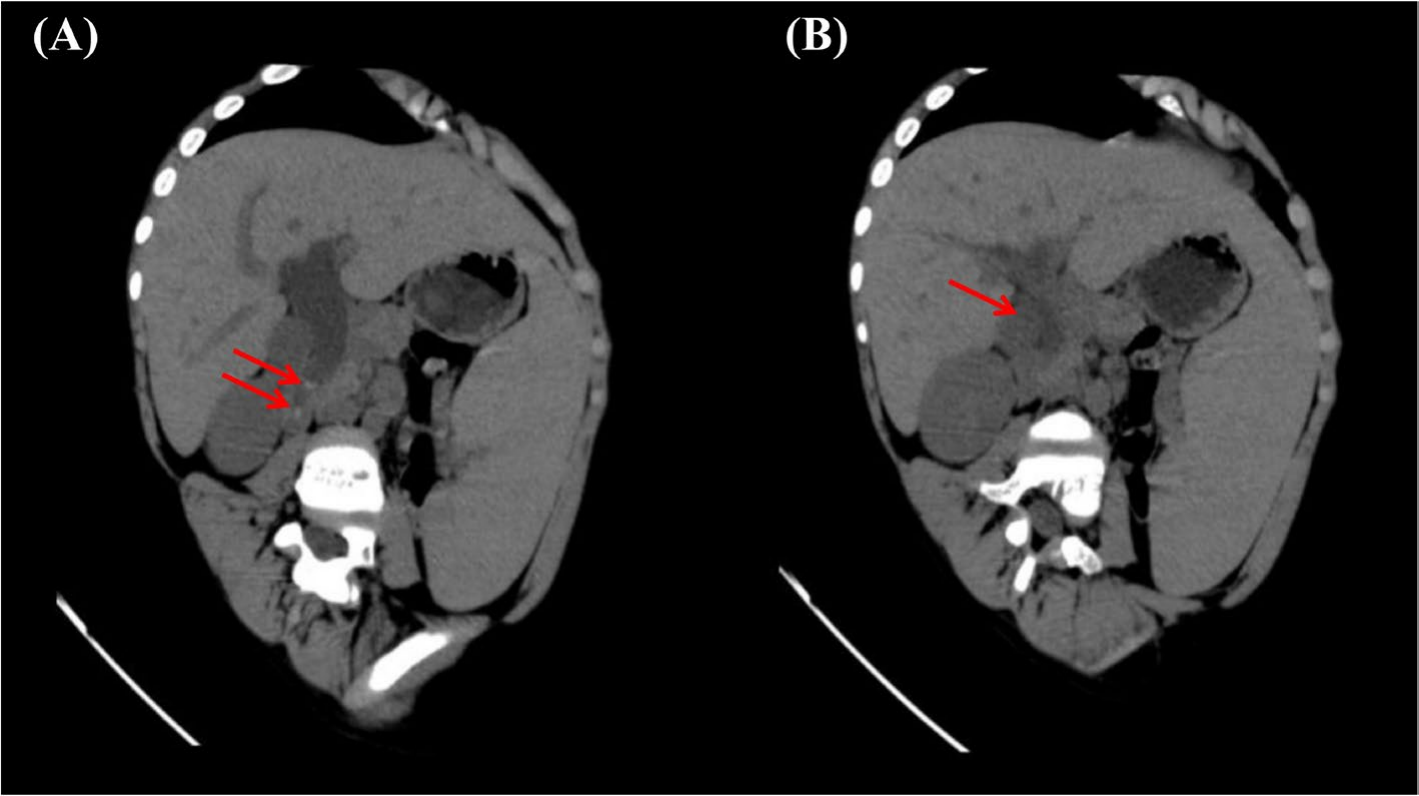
CT scan findings of the abdomen for this patient. A High-density shadow of the lower part of the common bile duct shown by the red arrow is considered to be gallstones. B Dilated intrahepatic bile duct shown by the red arrow
